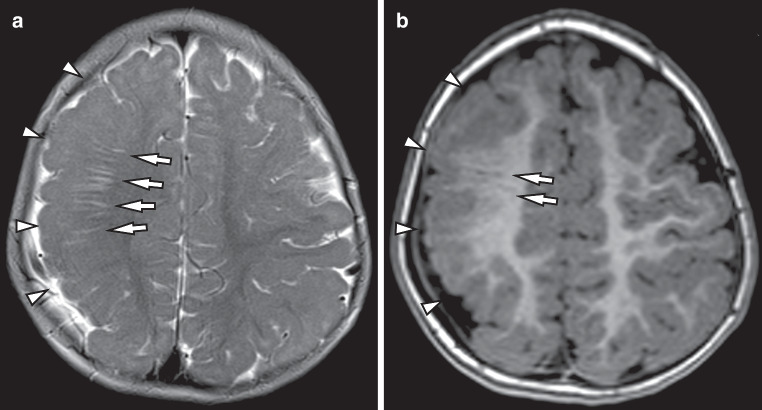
Enlarged perivascular spaces (*arrows*) underneath polymicrogyric cortex (*arrowheads*) in a 4 year old female patient with frontal and parietal lobar polymicrogyria (*arrowheads*). a Axial T2 weighted MR image. b Axial T1 weighted MPRAGE (magnetization prepared rapid acquisition with gradient echoes) image
